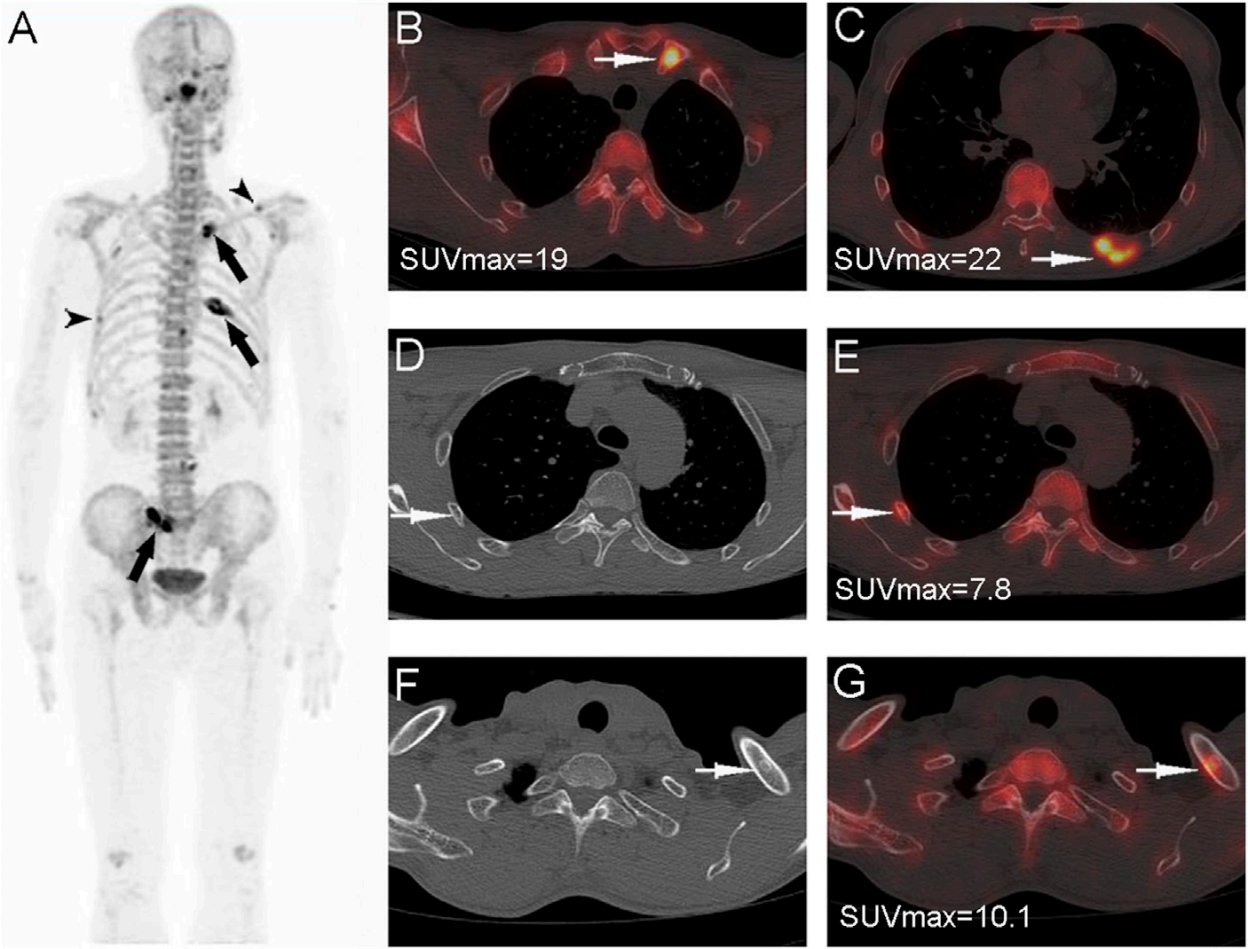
Nasopharyngeal carcinoma (T3N2M1) in a 55-year-old male patient. Whole-body PET MIP (A) shows multiple abnormally ^18^F-NaF uptake in multiple ribs, the right sacroiliac joint, and the left clavicle; the SUVmax of the confirmed bone metastases in the left clavicle (B), PET/CT fusion, arrows and the left 8th posterior rib (C), PET/CT fusion, arrows is significantly higher than that in the right rib (D,E), arrows and the left clavicle (F,G), arrows for benign lesions (non-fracture, non-osteophytes).
